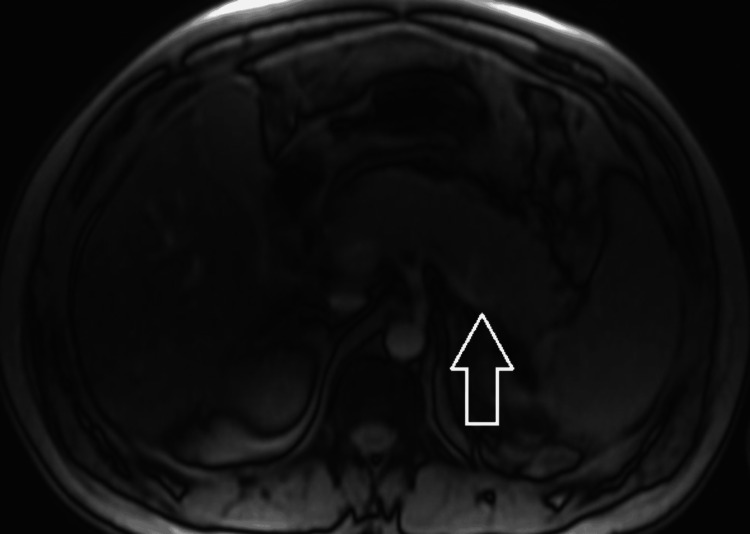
MRI/MRCP of the abdomen during early hospitalization Representative axial MRI/MRCP image obtained during early hospitalization. The arrow indicates an area of peripancreatic inflammatory change. MRCP, magnetic resonance cholangiopancreatography
